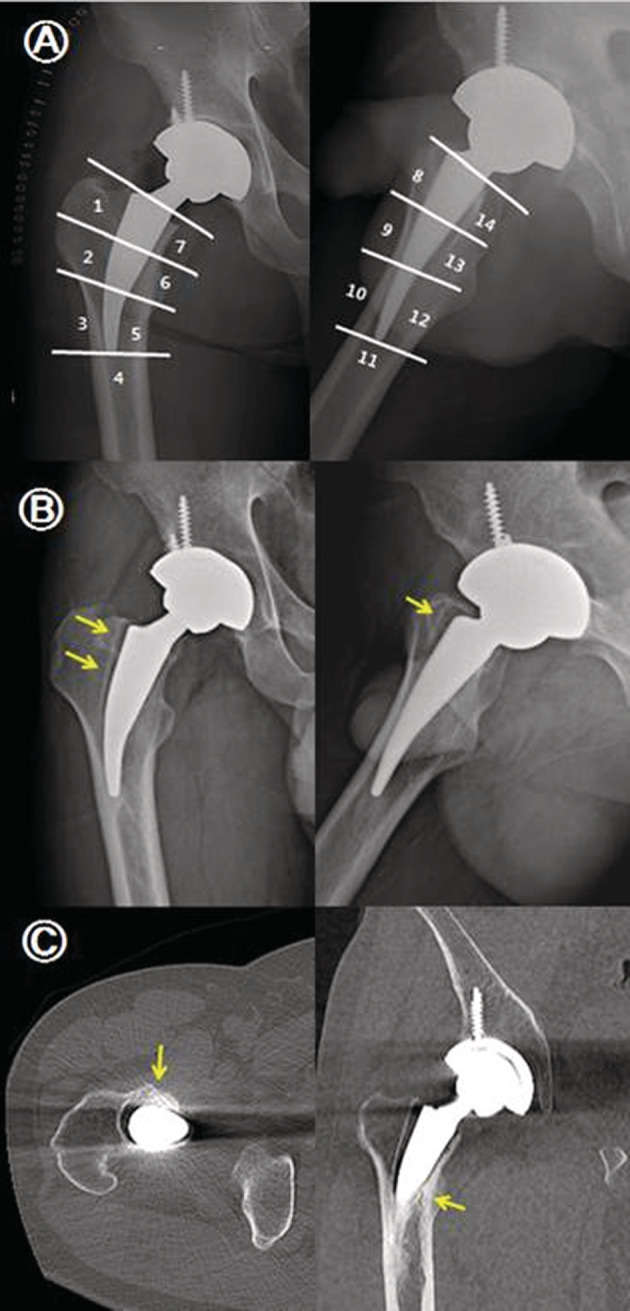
(A) Immediate postoperative radiograph of a 38‐year‐old man with avascular necrosis of the femoral head and definition of Gruen's periprosthetic zones. (B) Radiographs at 42 months postoperatively with pedestal formation at the distal smooth portion radiolucent line at zones 1, 2, and 8. (C) Axial and coronary CT scan images showing progression of osseointegration at sites other than osteolysis (arrow).
